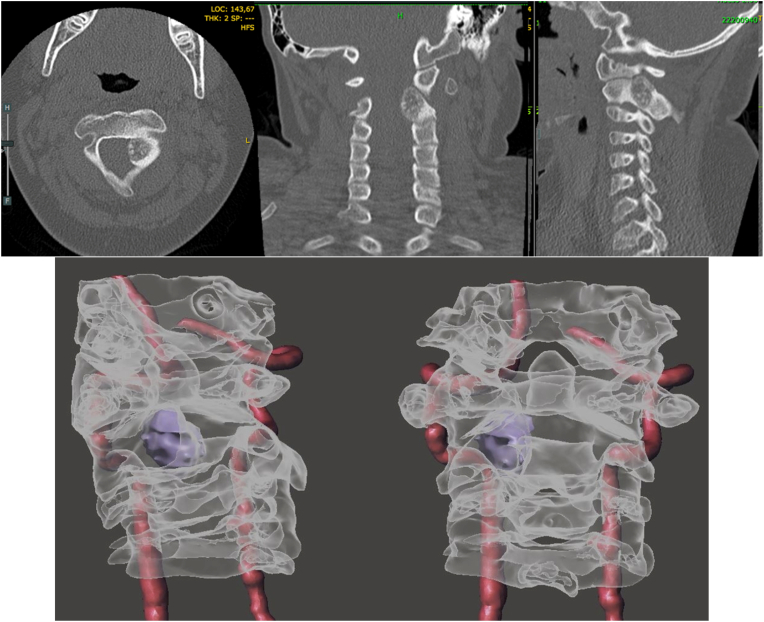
a) Axial, coronal, and sagittal CT bone window images showing a tumoral lesion in the left lamina of C2. b) Three-dimensional angio-CT reconstruction demonstrating the close relationship between the tumor and vascular structures.
